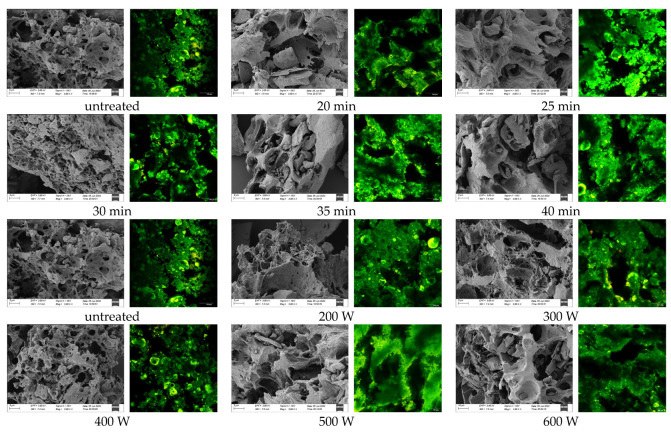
Effects of different ultrasonic treatments on SEM images of POPG: the left is the SEM image and the right is the CLSM image of each sample.
